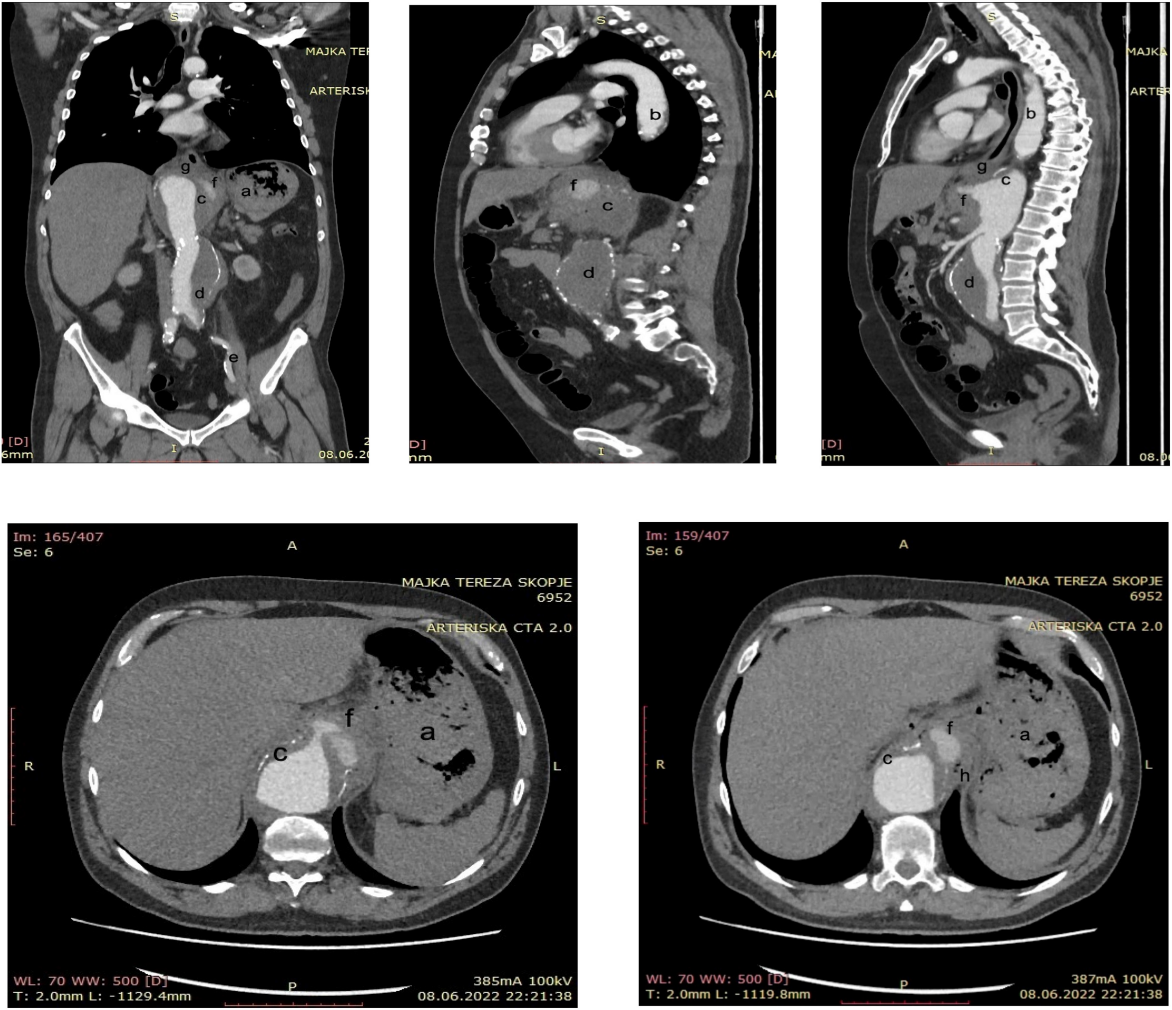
Thoracic and abdominal CT scan presenting the aneurysm of the thoracic and abdominal aorta propagating toward the left common iliac artery, mural thrombosis, and active contrast extravasation with air inclusions in the mediolateral segment of the extravasation. (a) Gastric content, (b) aneurism of the thoracic aorta, (c) suprarenal abdominal aorta, (d) infrarenal abdominal aorta, (e) aneurism propagating to the left common iliac artery, (f) contrast extravasation, (g) distal esophagus, and (h) air bubbles.
